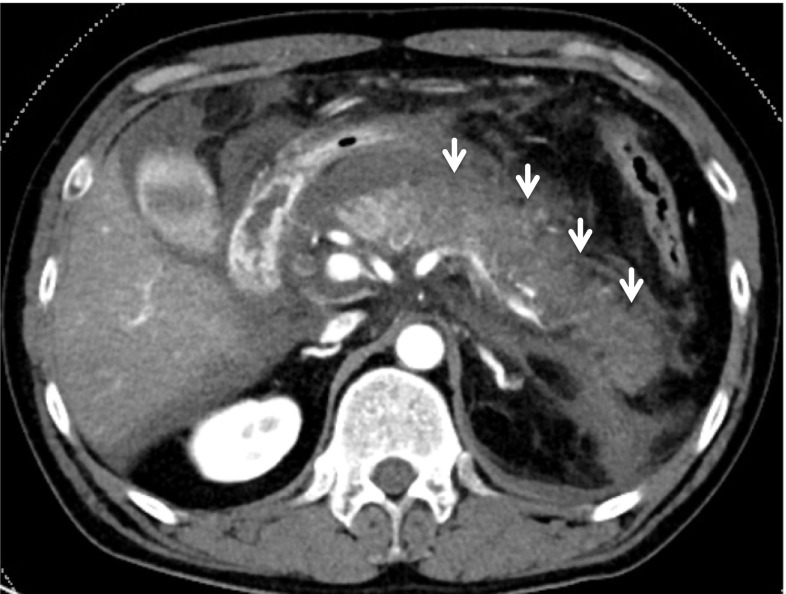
A contrast-enhanced CT image obtained within 48 h after the onset of AP. A CT image of a 46-year-old man shows LEPP (arrows) in the pancreatic body and tail with acute peripancreatic fluid collection. Imaging findings of this patient satisfied the inclusion criteria of this trial
